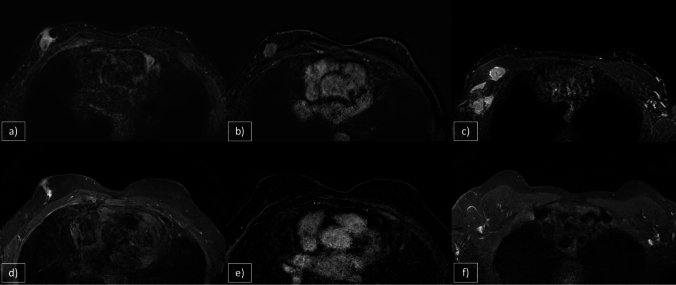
Male patient presented with bilateral gynecomastia and IDC on the right, deemed a candidate for neoadjuvant chemotherapy. Staging breast MRI revealed a hyperintense lesion on STIR sequences (a) and early enhancement on T1-CE images (b), accompanied by multiple ipsilateral axillary and retropectoral lymphadenopathies (c). Following six months of neoadjuvant chemotherapy, the patient demonstrated an excellent therapeutic response, (d) characterized by the absence of mass enhancement on post-contrast imaging (e). Additionally, no residual axillary lymphadenopathies were identified (f)
